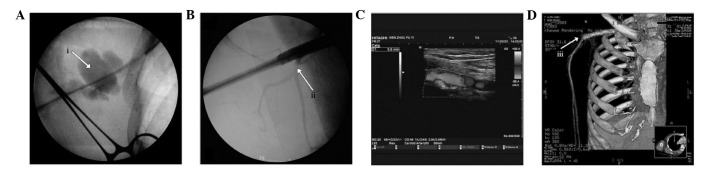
(A) CT scan of the injured region. Arrow i: contrast medium leaked from the proximal segment of the right axillary artery and the distal vessels were not visualized. (B) C-arm fluoroscopy-guided intraoperative arteriography. Arrow ii: the contrast medium passed normally through the proximal axillary artery and the distal and collateral vessels were clearly visualized. (C) B-mode ultrasonography at 6 months after surgery. The stent was patent, with no thrombus and slight intimal hyperplasia (2.7 mm) was present at the widest part of the inner wall. Blood flow was slightly reduced compared with the contralateral side. (D) CT arteriography at 6 months after surgery. Arrow iii: CT arteriography with three-dimensional reconstruction and distal vessel reconstruction, showing that the stent was correctly positioned and patent, with no distortion or deformation.
